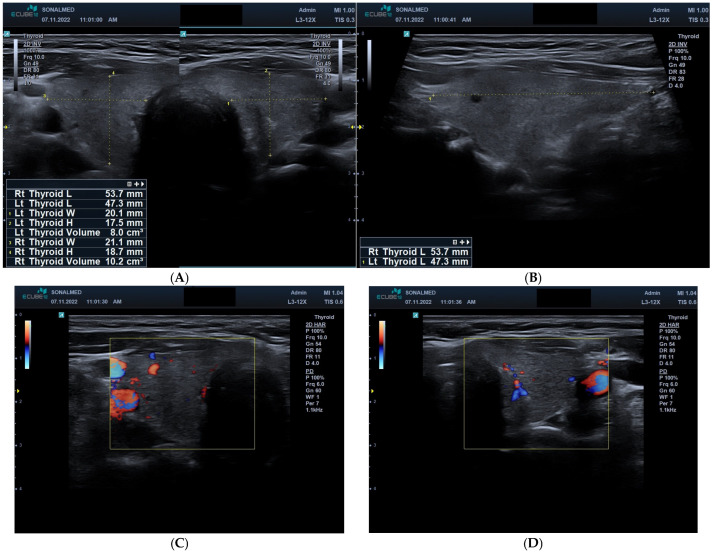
(A) Baseline transverse ultrasound image of the thyroid gland prior to sunitinib therapy. Grey-scale imaging shows both thyroid lobes with normal morphology and homogeneous parenchymal echotexture. Measurements indicate a left lobe volume of 8.0 cm^3^ and a right lobe volume of 10.2 cm^3^, consistent with values within the normal range. No focal lesions or structural abnormalities are visible. (B) Baseline transverse grey-scale ultrasound image of the thyroid gland. The image demonstrates both thyroid lobes in transverse view, showing normal parenchymal echogenicity and homogeneous internal structure. Measured thyroid lobe lengths (right: 53.7 mm; left: 47.3 mm) fall within the expected physiological range. No focal abnormalities or structural distortions are observed. (C) Baseline transverse colour Doppler ultrasound image of the thyroid gland prior to sunitinib therapy. The image shows a moderate number of intrathyroidal blood flow signals distributed focally within the thyroid parenchyma. Vascularisation is preserved, with no evidence of significant perfusion reduction or asymmetry between the lobes. (D) Baseline transverse colour Doppler ultrasound image of the thyroid gland prior to sunitinib therapy. Colour Doppler imaging demonstrates preserved intrathyroidal blood flow signals, visible as focal areas of vascularisation within the parenchyma. No significant perfusion defects or vascular asymmetry are observed, indicating normal baseline thyroid vascularity.
